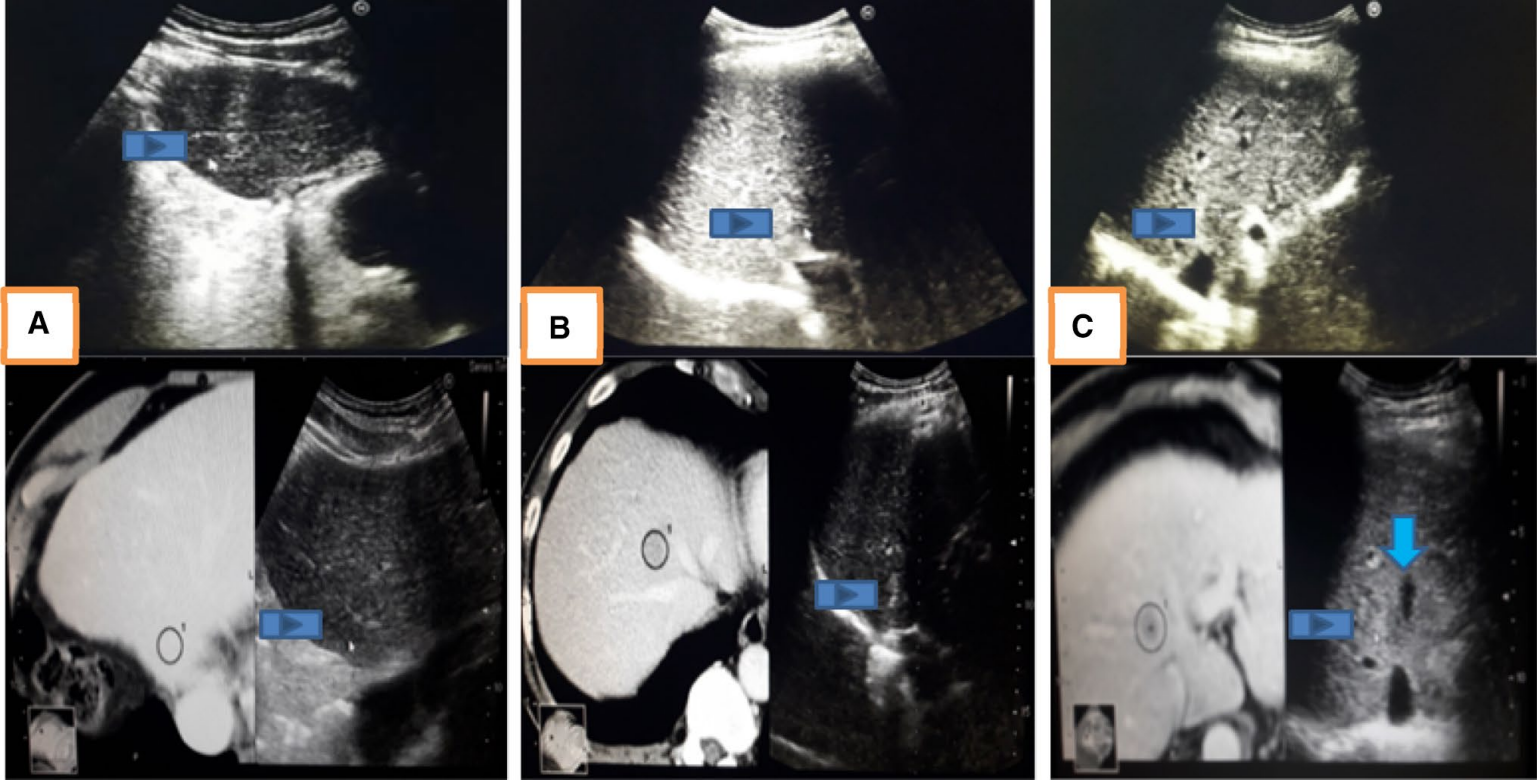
The images above show the tumor visibility under ultrasound and under RVS. a Conspicuous S4 tumor with visible margin and echogenicity b Inconspicuous S8 tumor without visible margin c Inconspicuous S8 tumor without visible margin, beside middle hepatic vein
